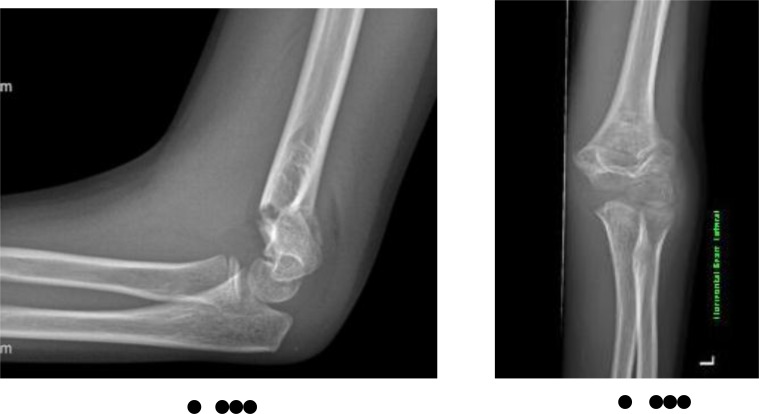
AP and lateral radiographs of Wilkin’s-modified Gartland Grade 2a supracondylar humeral fracture.
